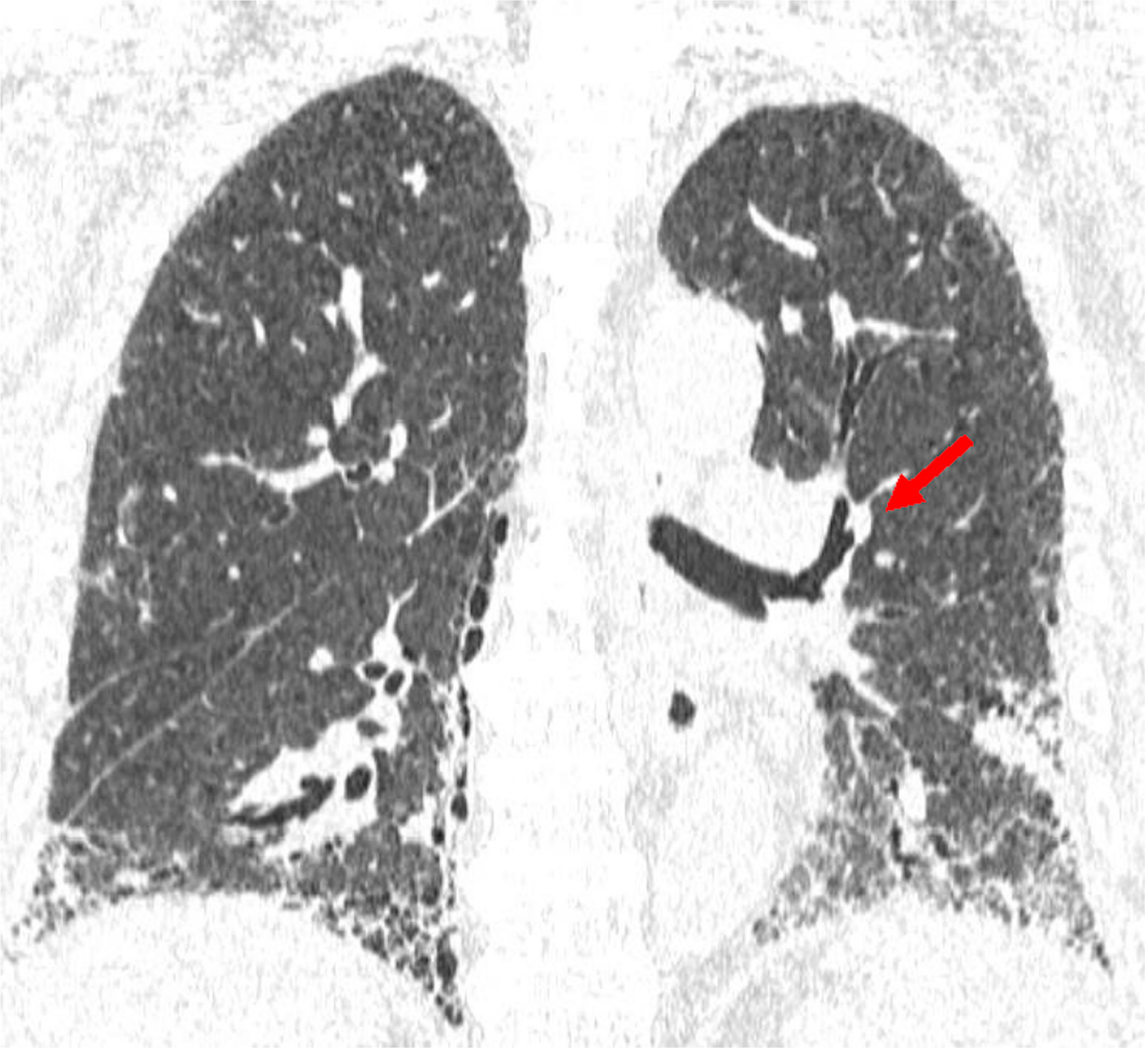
Computed tomography findings before transbronchial cryoablation. Computed tomography shows interstitial pneumonia with a subpleural and basal predominance, as well as a nodule located in the superior segmental bronchus of the left lung (arrow).
